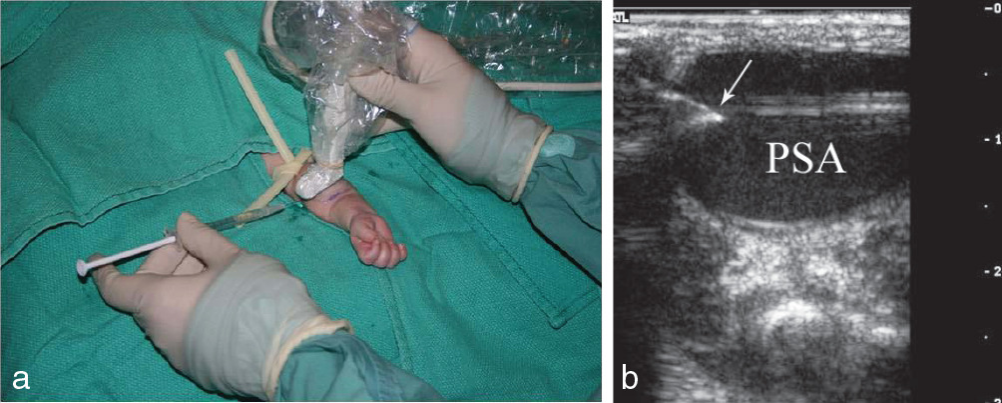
(a) Thrombin was injected into the lesion under real-time sonographic guidance and sterile conditions with a 25-gauge needle. (b) Transverse sonographic view showing the needle (arrow) positioned in the sac of the PSA prior to the injection. PSA, pseudoaneurysm.
